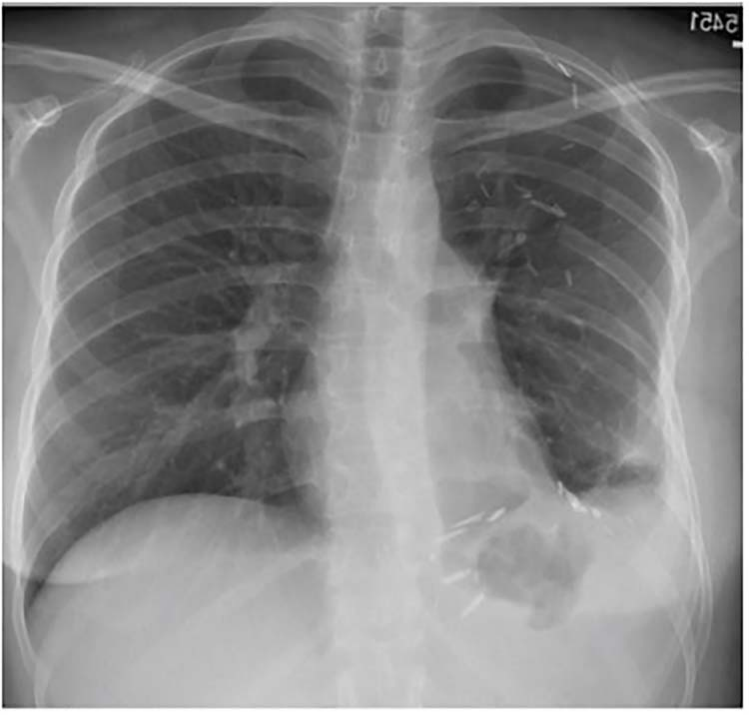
Frontal chest radiograph 2 months after resection with near-complete left lung expansion.
